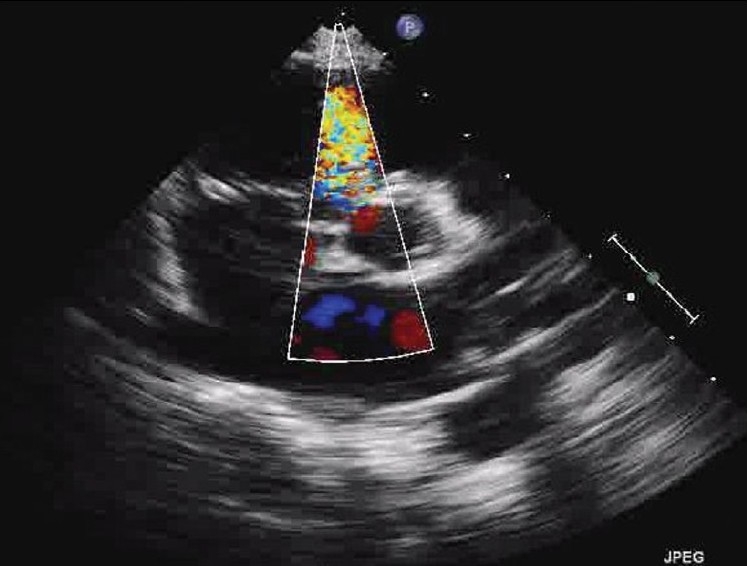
TTE image parasternal short axis view showing small perimembranous VSD with left to right shunt
